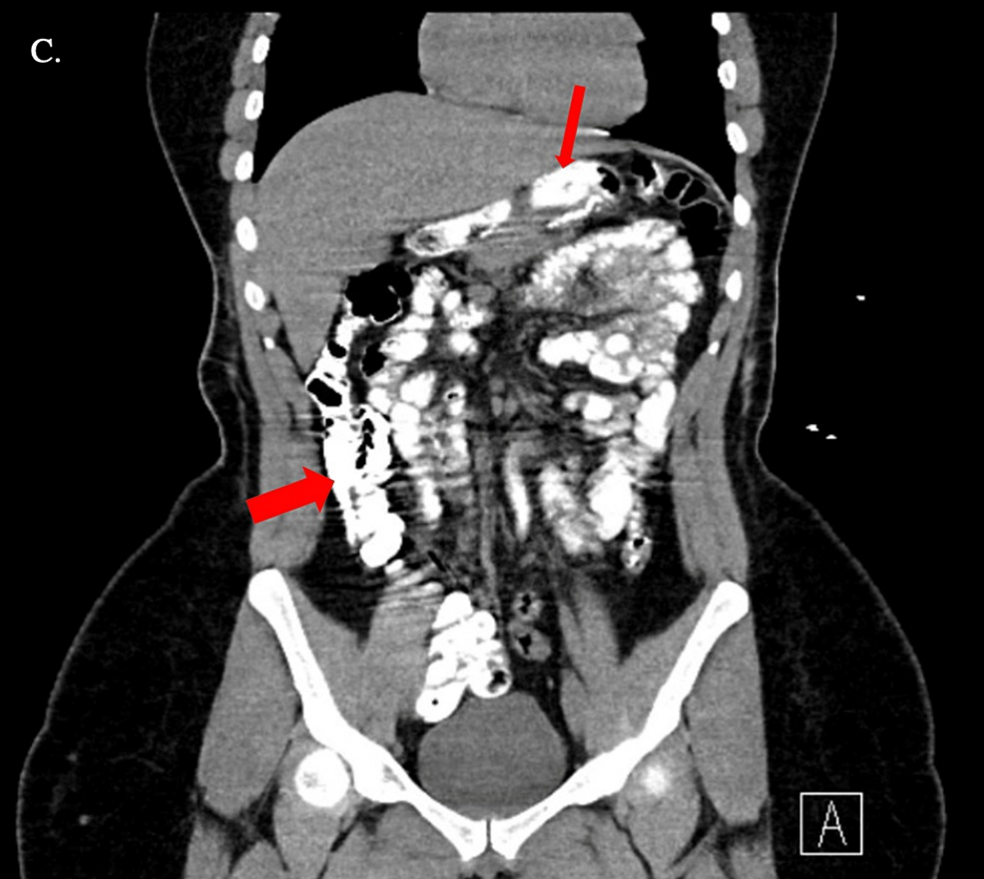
CT abdomen and pelvis with oral contrast (frontal view) Frontal view of the abdomen demonstrating post-surgical gastric sleeve with contrast in the gastric sleeve (thin arrow) and bowels (thick arrow).
